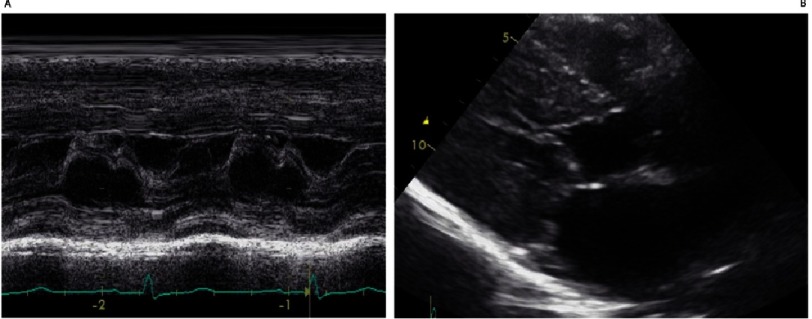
A) M-Mode echocardiography: Classical systolic anterior movement (SAM) of the mitral valve causing obstruction. B) B-Mode Echocardiography: Paraesternal longitudinal axis showing contact between interventricular septum and the anterior leaflet of the mitral valve in the beginning of systole.
